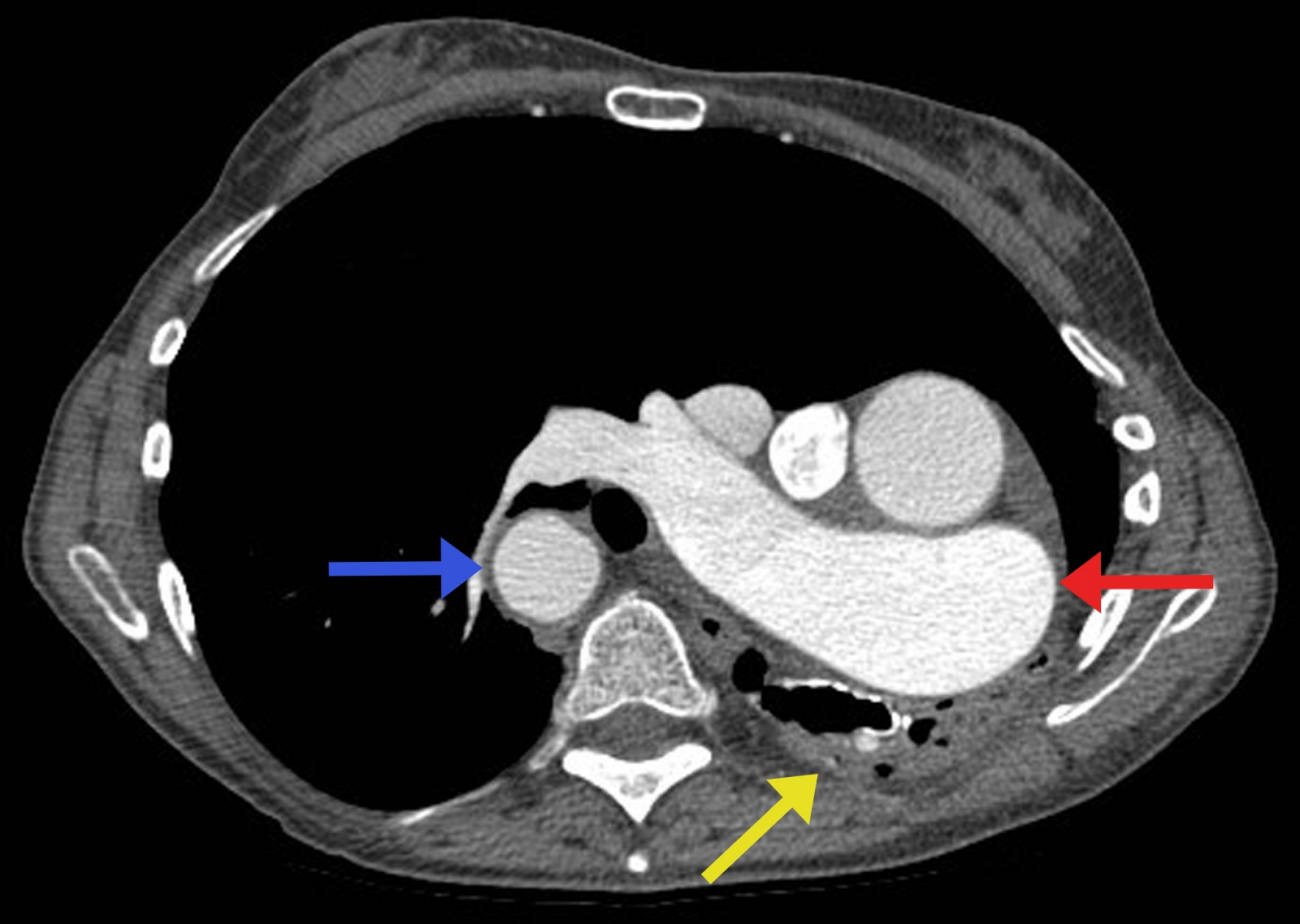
Contrast-enhanced CT chest, axial image. Pulmonary trunk emerges from the right ventricular outflow track and entirely feeds deoxygenated blood into an enlarged right pulmonary artery (red arrow). The left lung is almost entirely hypoplastic with a few dilated bronchi/bronchioles and small caliber vessels (yellow arrow). Incidental note is made of a right-sided aortic arch (blue arrow).
